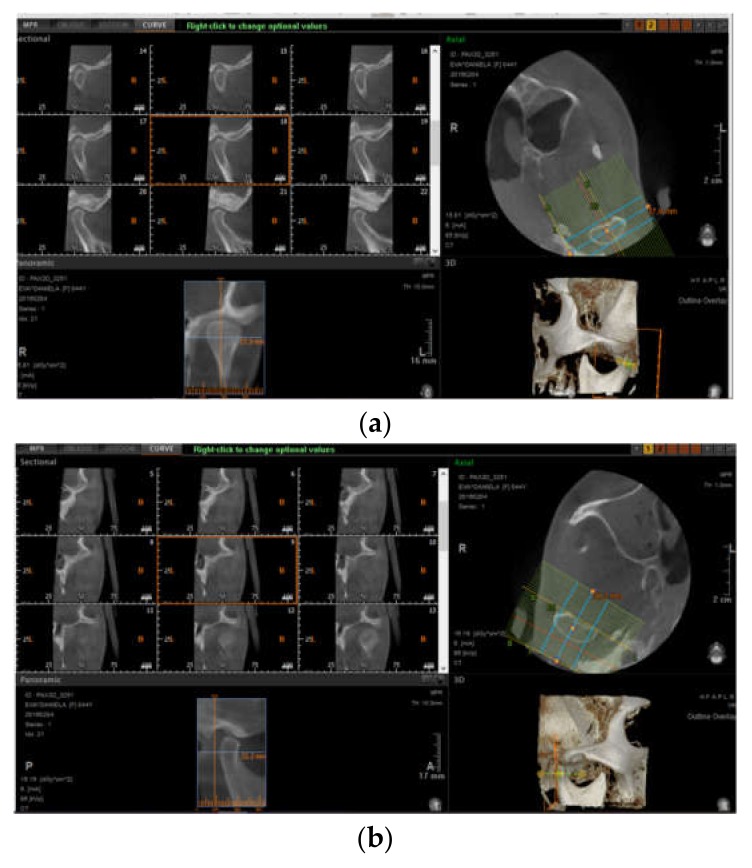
CBCT aspects of the (a) right and (b) left TMJ.
